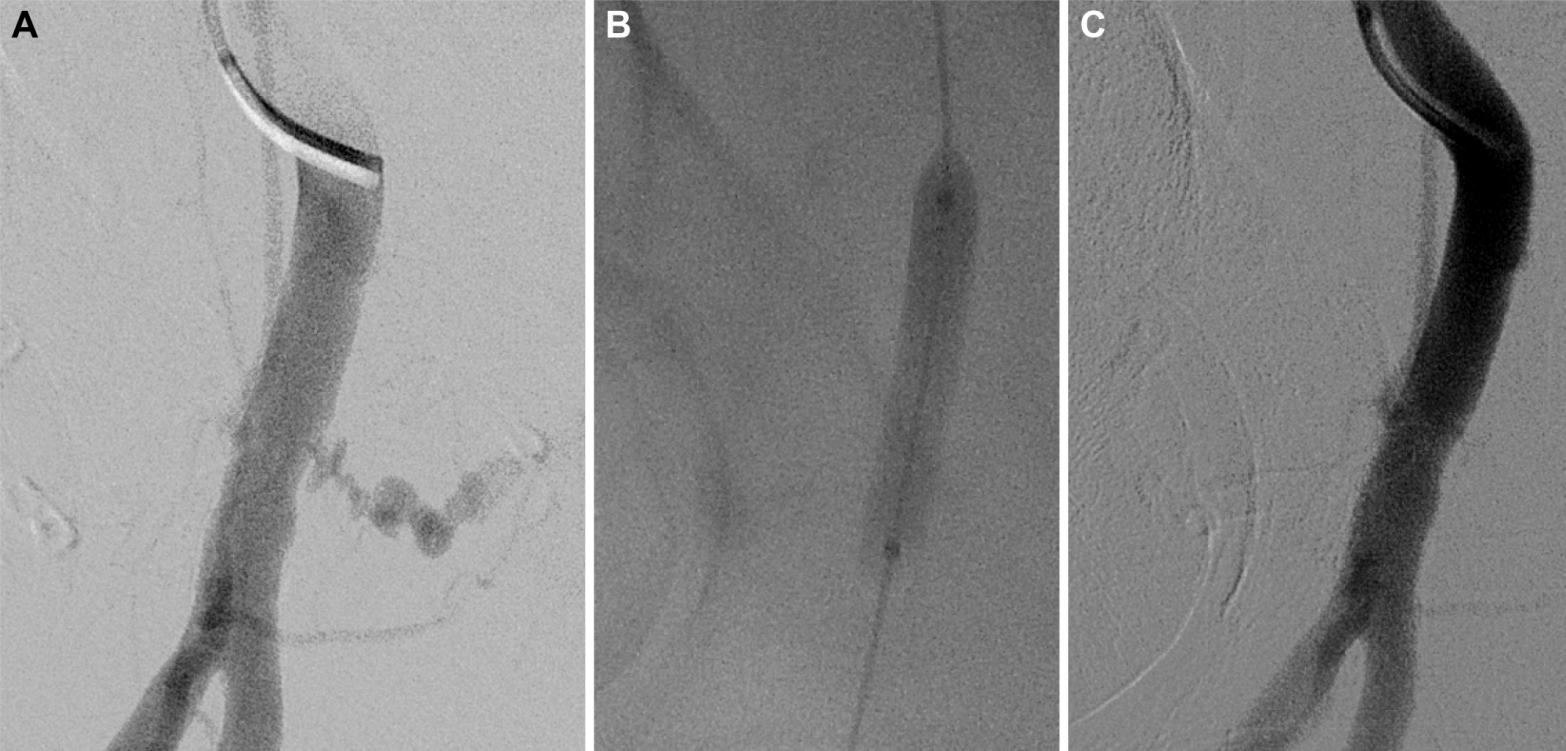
(a) Iatrogenic pseudoaneurysm after failed manual compression. (b) After placing an angioplasty balloon (6 mm in diameter) from a contralateral femoral approach and inflation, thrombin injection was performed under ultrasound guidance. (c) Angiography showing successful thrombosis of the pseudoaneurysm after percutaneous thrombin injection.
